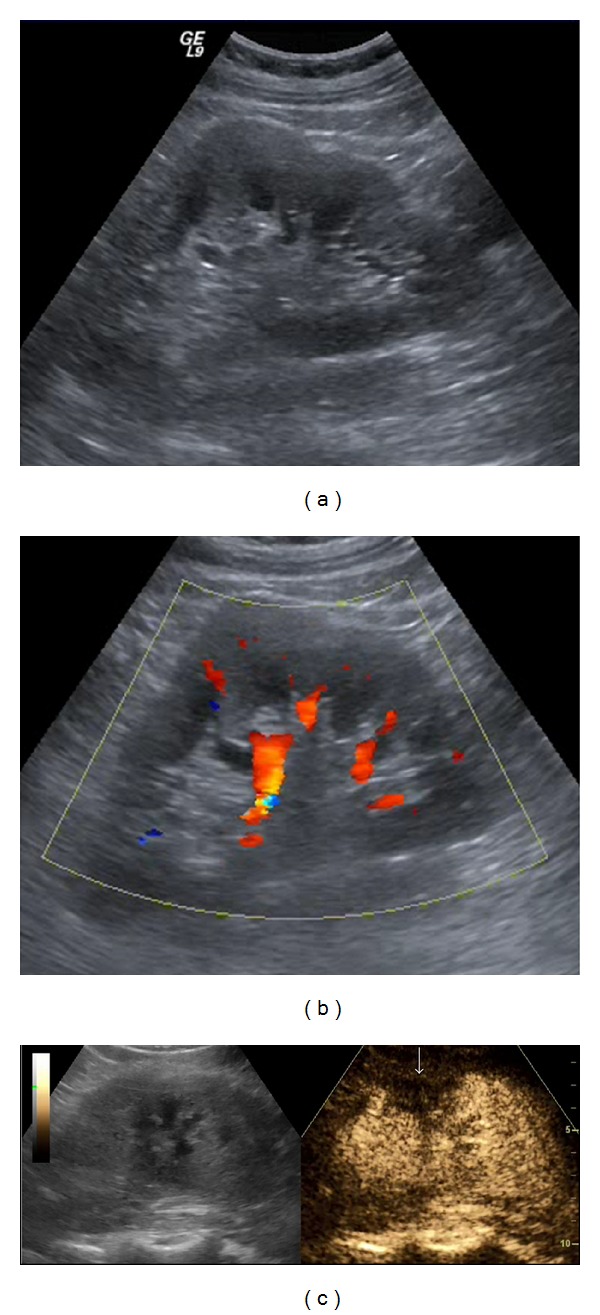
Kidney infarct: B-mode (a) and Colour Doppler (b) US detect no abnormality in the left kidney. On CEUS however (c) a triangular peripheral enhancement defect is evident (arrow).
